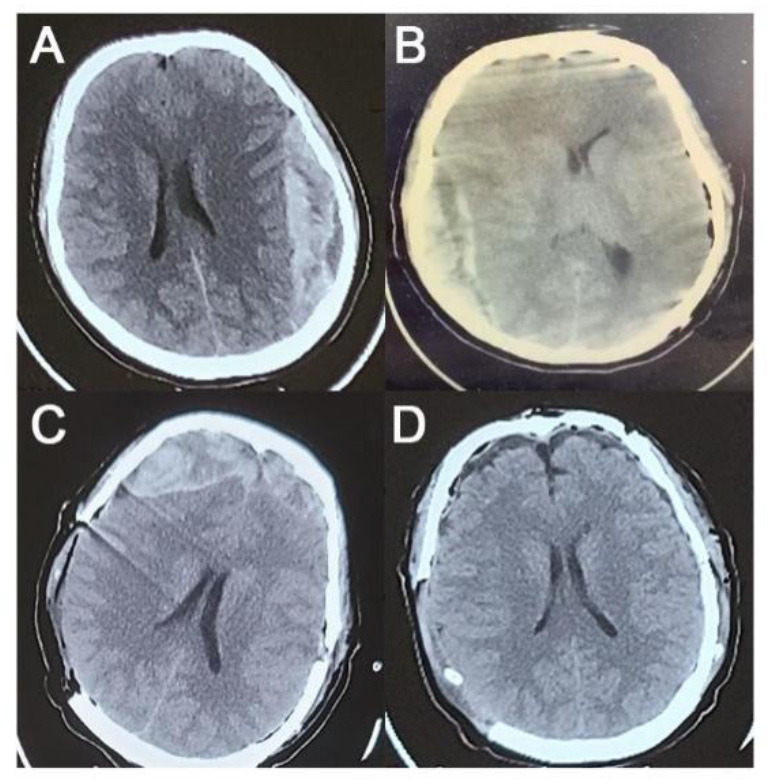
Preoperative and postoperative CT images. (A) Preoperative CT. (B) CT scan after the first operation. (C) CT scan after the second operation. (D) CT scan after the third operation.
